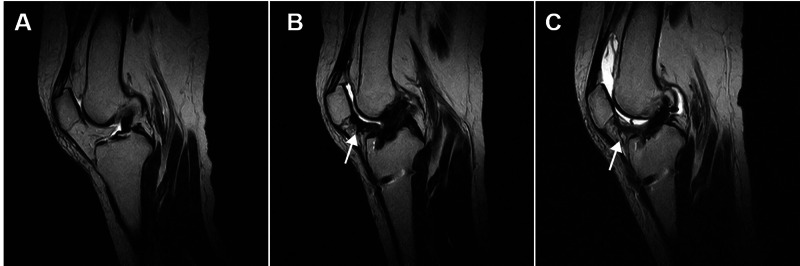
Magnetic resonance imaging (A) Pre-arthroscopic anterior cruciate ligament reconstruction -- indicates anterior cruciate ligament tear. (B) Post-surgery -- the anterior cruciate ligament continuity is confirmed. The scar of the infrapatellar fat pad (arrow). (C) Ten months after surgery -- the anterior cruciate ligament is not re-tearing. The scar of the infrapatellar fat pad (arrow).
